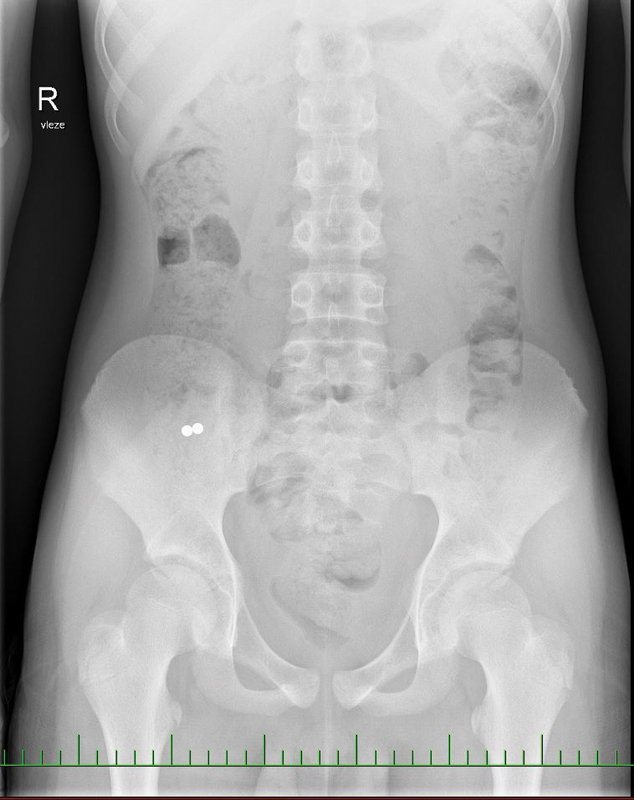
Abdominal X-rays contrasting oval MFBs in the right hypogastrium. MFBs, magnetic foreign bodies.
